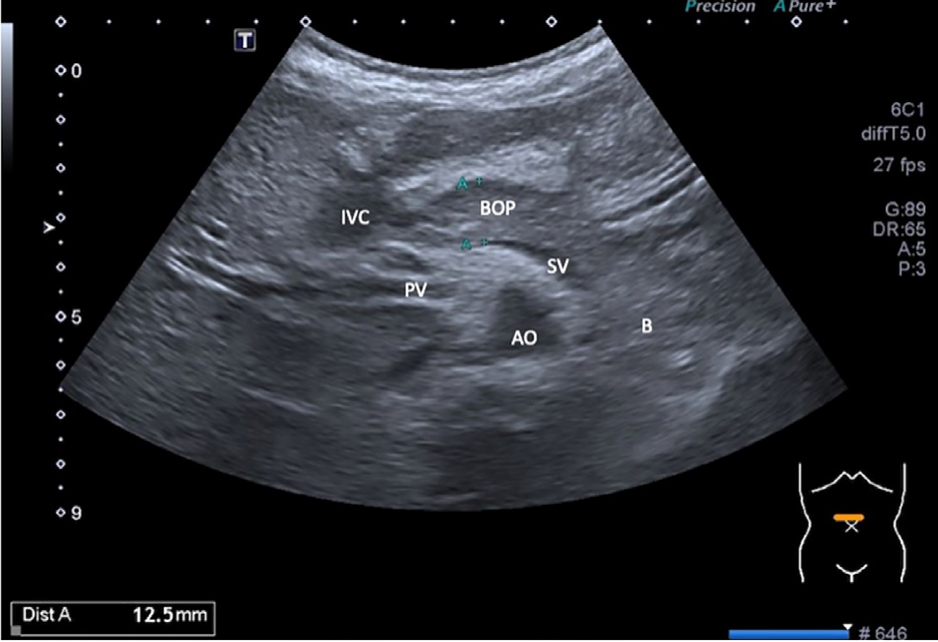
Transabdominal ultrasound mid axial plane at supraumbilical region showing homogenous mild hyperechoic appearance of pancreas which is normal for age and no evidence of laceration in the visualised neck and body of pancreas. The tail of pancreas is not visualised due to bowel gas obscuration. No peripancreatic free fluid. The rest of abdominal organs are unremarkable (not shown). AO: abdominal aorta, B: bowel, SV: splenic vein, PV: portal vein, IVC: intrahepatic inferior vena cava, BOP: neck and body of pancreas
